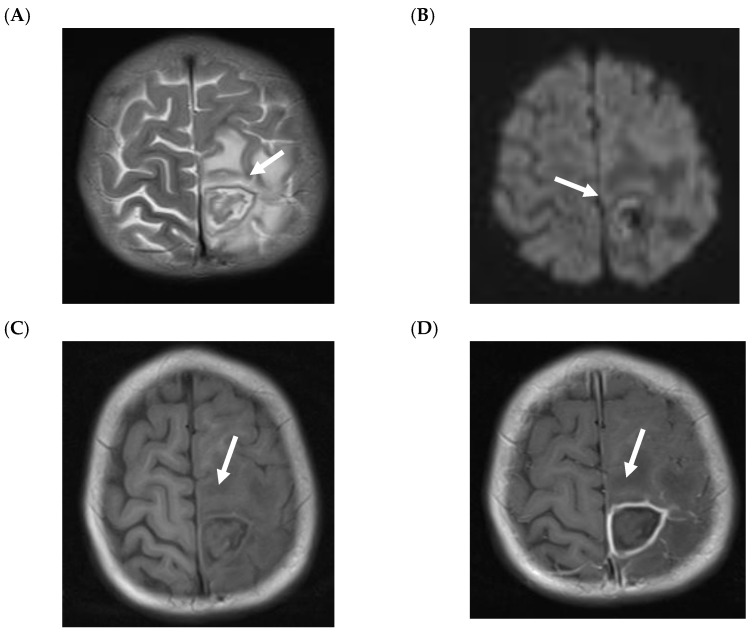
Fungal abscess in a 3-year-old girl with acute lymphoblastic leukemia and proven central nervous system invasive mold infection. The patient is alive with residual right-sided weakness. Axial T2-weighted (A) shows a mass in the left central region, which is predominantly of low T2 signal intensity, centrally with hyperintense perilesional edema and a well-defined ring-like hypointense area (arrow). Diffusion-weighted image (DWI), b = 1000 s/mm^2^, shows mainly restriction within the hypointense rim (B, arrow). Axial unenhanced T1-weighted shows a slight rim-like high signal intensity probably due to the presence of iron, manganese or methemoglobin (C, arrow). T1 after contrast shows a strong ring enhancement (D, arrow).
